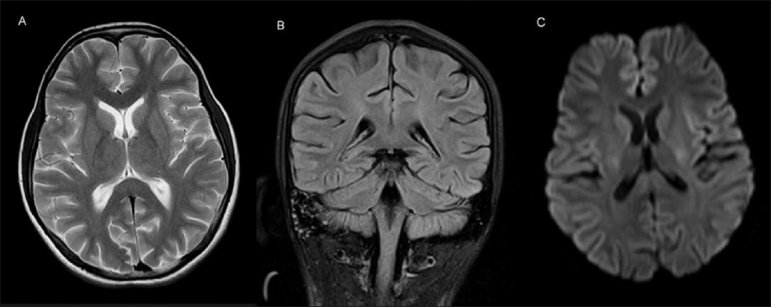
Axial T2W (A), coronal FLAIR (B), and diffusion-weighted (C) images show complete disappearance of the lesion seen in Figure 1
